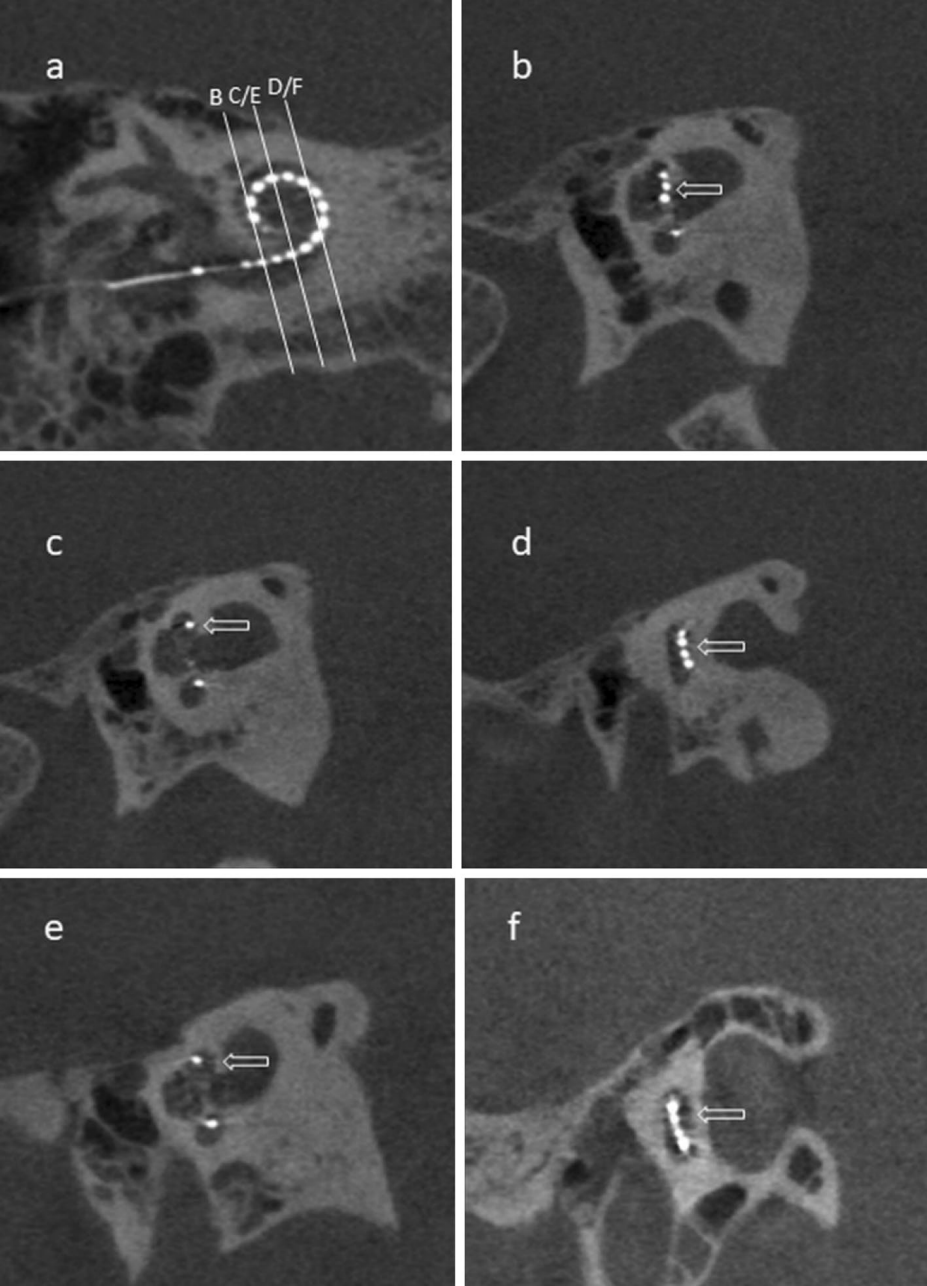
Assessment of scalar translocation. (a) Oblique coronal cone beam CT image showing the location of sagittal oblique sections within the descending segment (B), superior and inferior segments (C,E) and ascending segment (D,F). (b–d) images demonstrate a scala tympani (ST) location of the electrode array throughout without crossing. Arrows in (b–d) indicate the electrode array in the posterior aspect of the descending turn, superior segment and ascending segments respectively. Note the electrode array is also depicted within the posterior aspect (ST) of the inferior segment in (c). (e,f) images demonstrate crossing of the electrode array from the ST to scala vestibuli (SV) compartments. Arrow in (e) indicates the anterior position (SV) of the electrode array whereas the electrode array in the inferior segment is posteriorly positioned (ST). Arrow in (f) shows the electrode array passing from posteriorly to anteriorly within the ascending segment.
